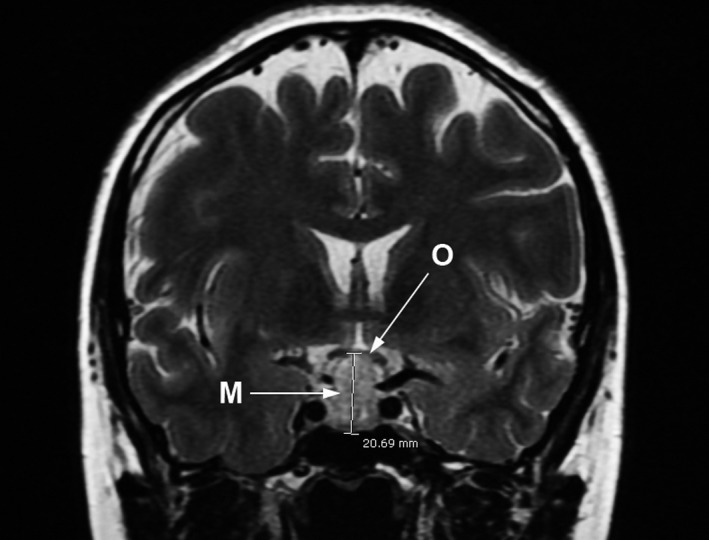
T2‐MRI Pituitary coronal scan at 32 wk of gestation: macroadenoma (M) with significant compression of optic chiasm (O)
